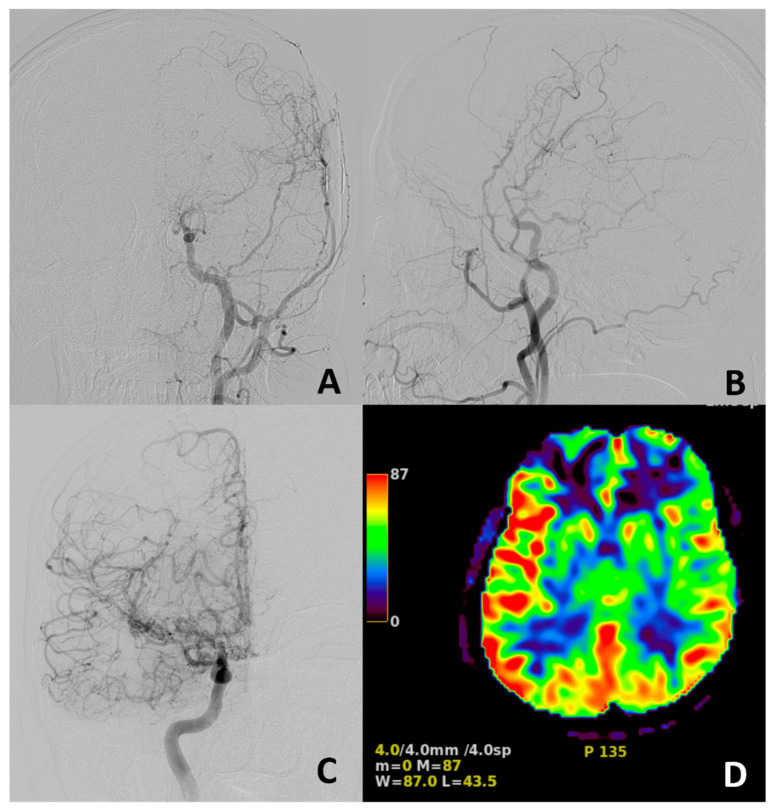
A 13-year-old girl with left-sided moyamoya disease treated with indirect revascularization. Follow-up DSA at 12 months (A,B) demonstrates Matsushima grade C collateral formation. (C) Right-sided Suzuki stage III disease. (D) ASL perfusion MRI demonstrating reduced cerebral blood flow in the left hemisphere.
